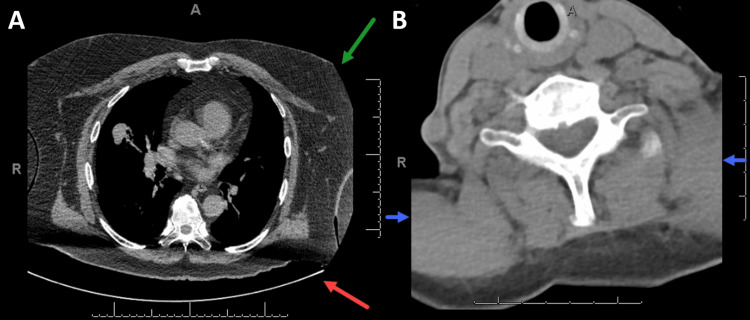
(A) Diagnostic CT scan of the chest showing a curved tabletop (red arrow) limiting reproducibility of patient position and narrow FOV cropping the body (green arrow) limiting the ability to use fields that pass through this area. (B) Diagnostic CT scan of the cervical spine showing a narrow FOV which crops the body (blue arrows) and prevents the ability to use parallel opposed fields. FOV: field of view
